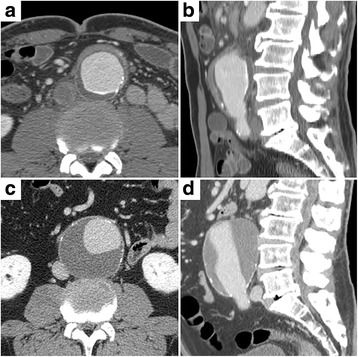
Contrast-enhanced CT (delayed phase) of IgG4-related perivascular lesion in a 72-year-old man. Walls of the abdominal artery showed thickening at diagnosis (a transverse plane; b sagittal plane). Steroid therapy reduced wall thickness but dilated the vascular lumen (c transverse plane; d sagittal plane)
